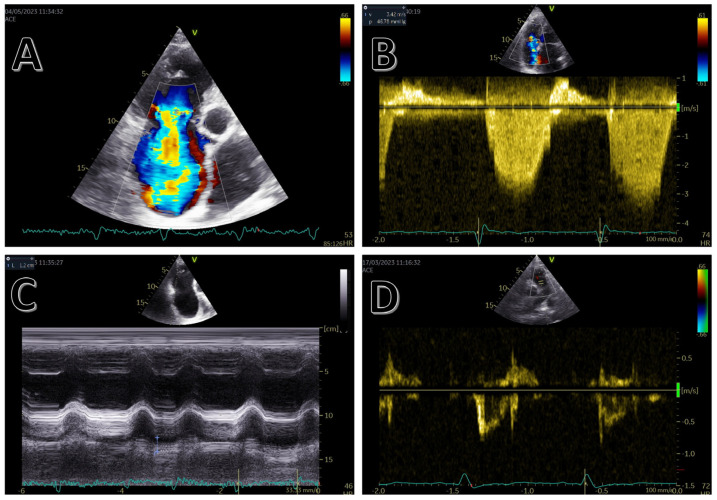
Echocardiographic markers of right ventricular impairment. (A) Significant tricuspid regurgitation. (B) High tricuspid gradient. (C) Right ventricular disfunction demonstrated by low TAPSE. (D) Short pulmonary acceleration time.
